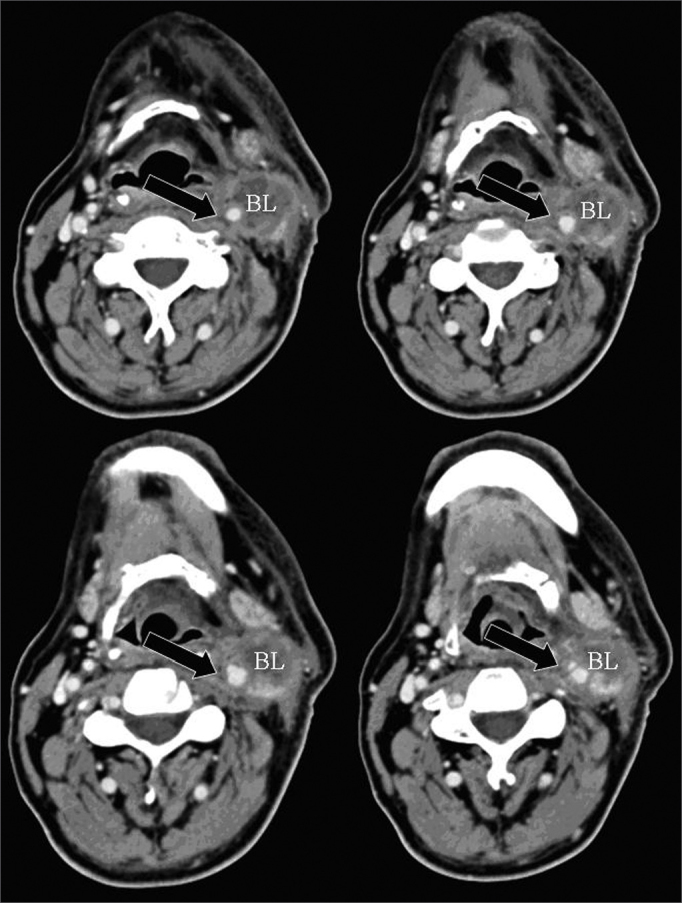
axial CT with endovenous contrast. Lymphadenomegaly / high jugular-carotid (level II) lymph node block (BL) to the right with a contact interface below 50% of the internal carotid artery circumference. CI: internal carotid artery; CE: external carotid artery.
